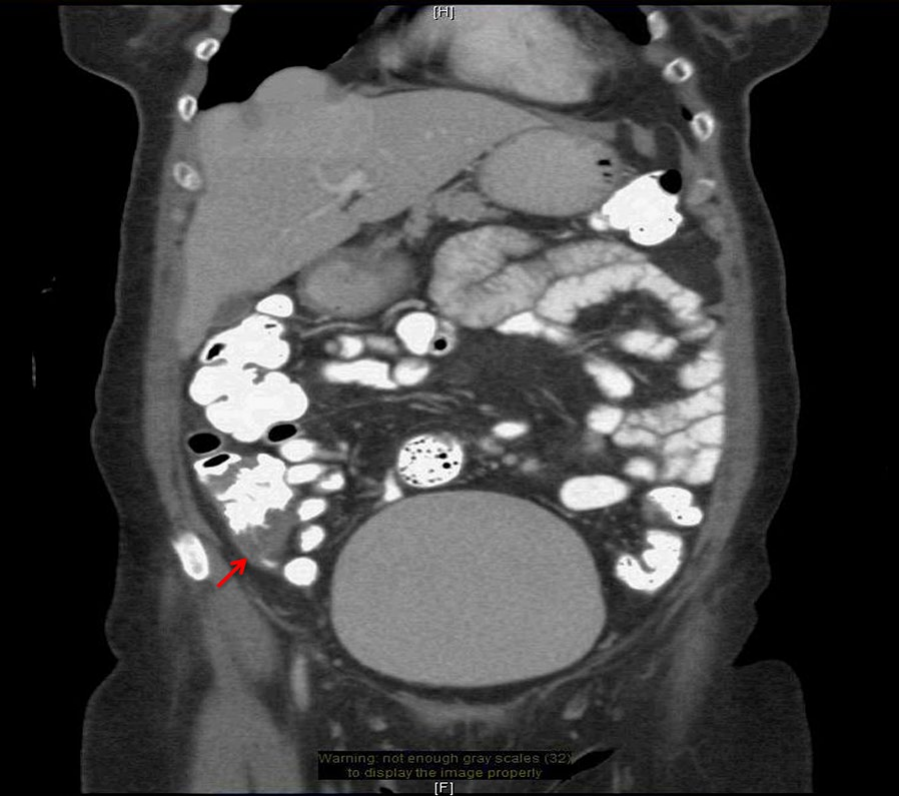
CT scan shows thickening and inflammatory change of a tubular structure extending from the base of the cecum, surrounding with inflammatory change
